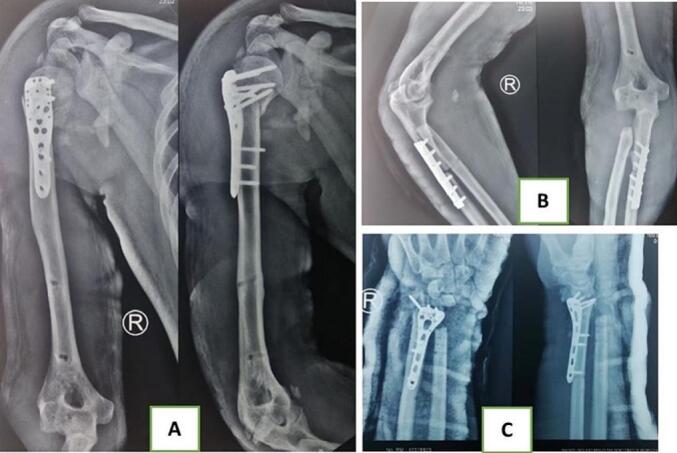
Postoperative radiographs after definitive surgical intervention; (A) humerus anteroposterior and lateral, (B) forearm anteroposterior and lateral, and (C) wrist anteroposterior and lateral.
